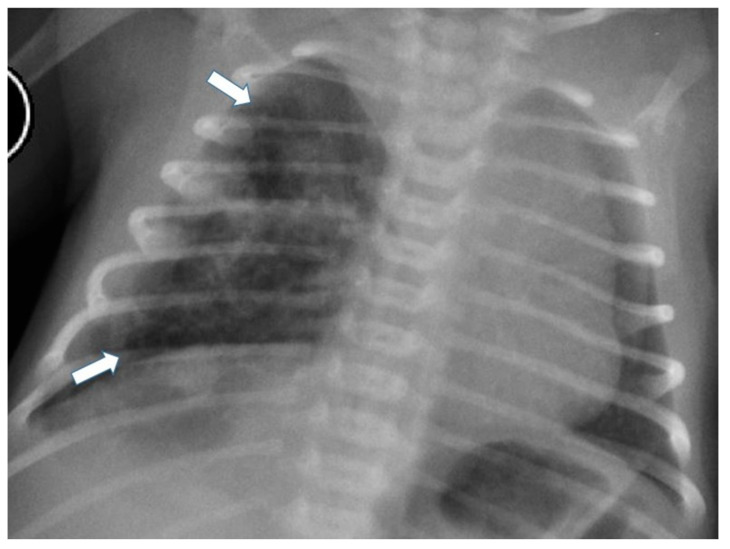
Chest radiograph of a newborn shows multiple small air-filled cysts in the right upper lobe and right lower lobe (arrows), suggesting a type 2 CPAM.
